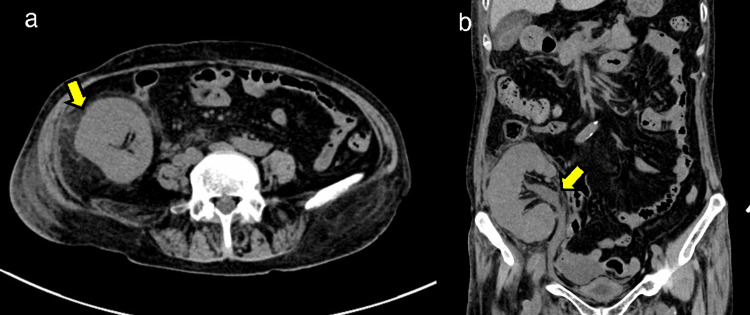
Abdominal computed tomography revealing swelling of the transplanted kidney, dilation of the ureter and renal pelvis, and perirenal fat stranding.
